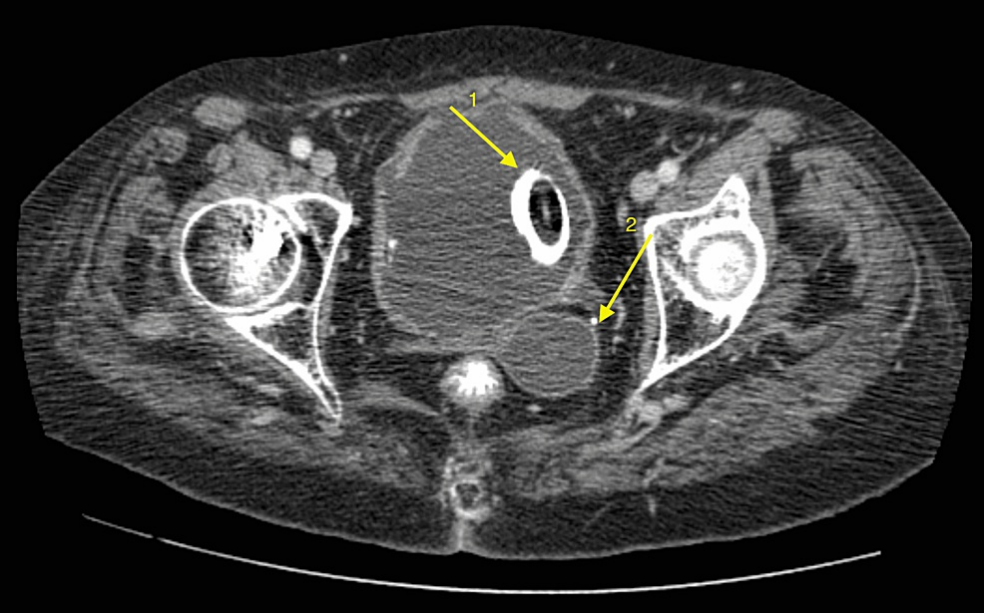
Computed Tomography of Bladder Foreign Body 1. Foreign body in urinary bladder encrusted; 2. Foley catheter in vagina
